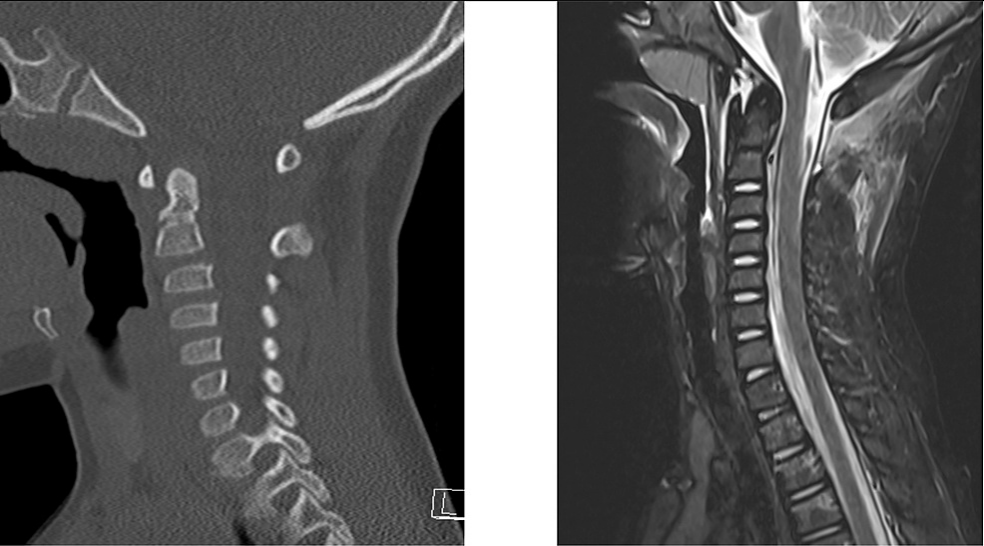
Preoperative CT (left) and MRI (right) scan CT imaging shows a widening of the predental area with no acute bony fracture. MRI shows a widening of the predental space and BDI. Disruption of the apical ligament of the dens and posterior ligamentous complex is apparent, along with a small prevertebral hematoma at C1-C2. BDI: basion-dens interval
